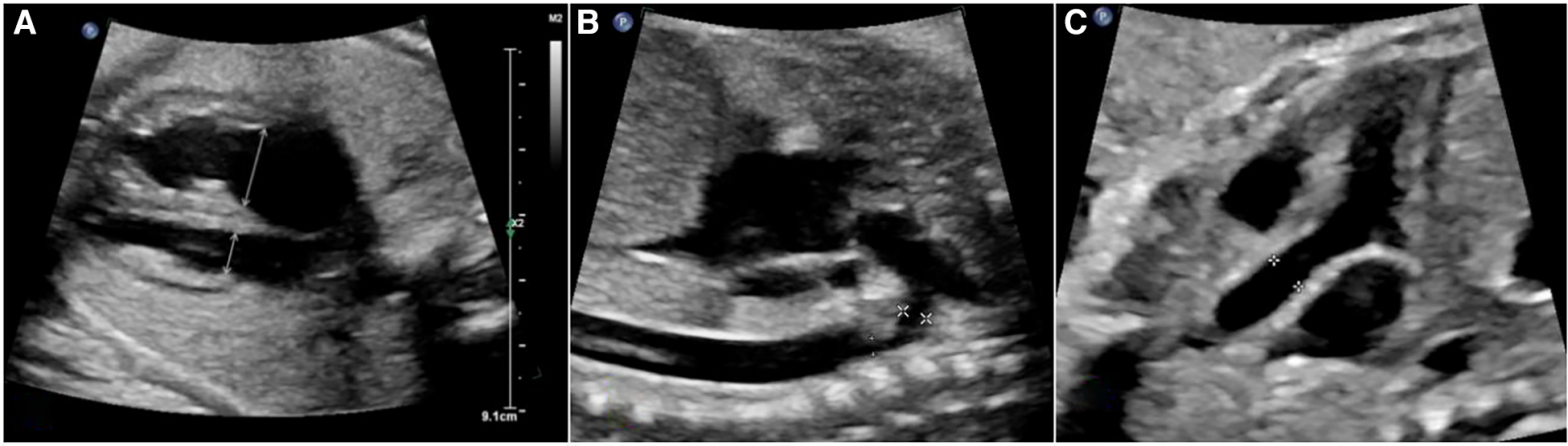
The assessments of aorta and cardiac structure based on fetal echocardiography. (A) four-chamber view of a fetus demonstrating ventricular size discrepancy and location where measurements of TVA and MVA were obtained. (B) The sagittal view of aortic arch in fetal echocardiography. The calipers show the location of measurement of inner diameter of tA was taken between origin of right brachiocephalic artery and left subclavian artery (
